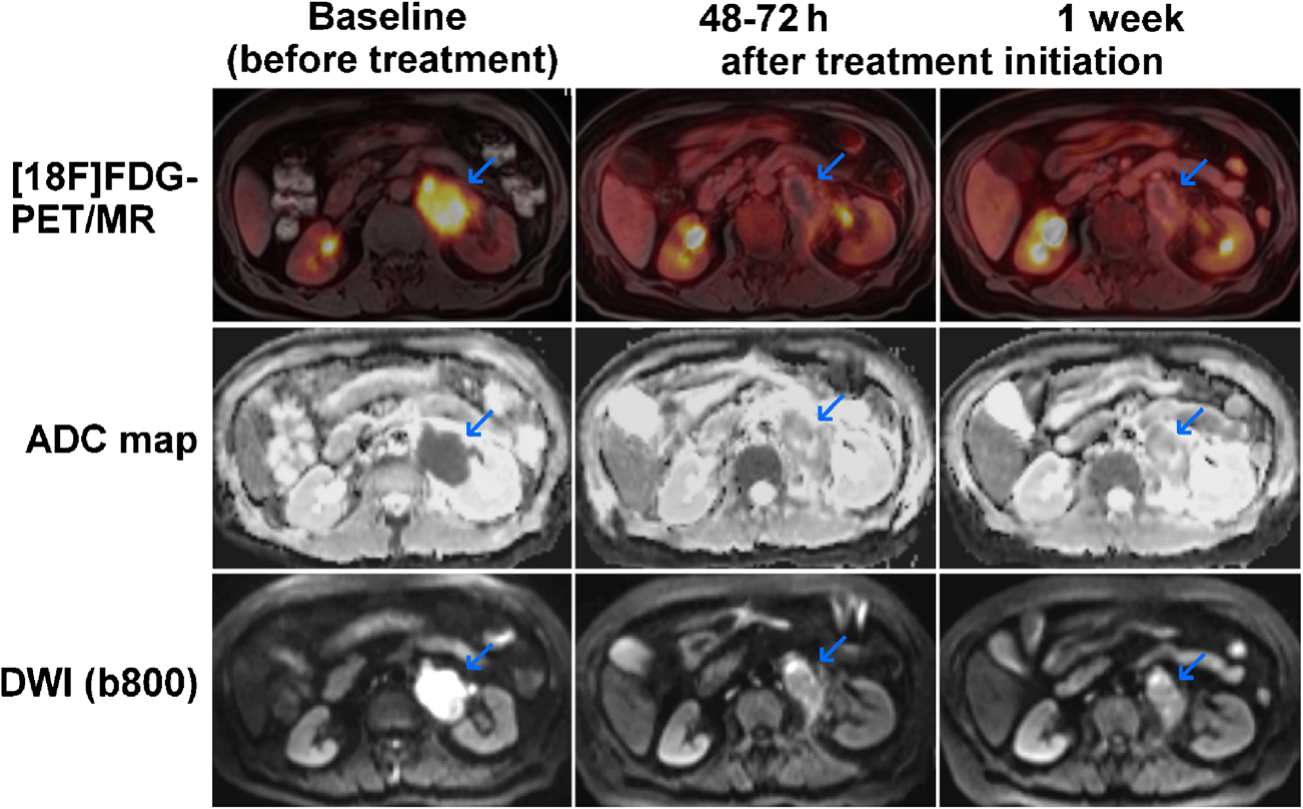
A 68-year-old woman with DLBCL of the left adrenal gland (*blue arrows*). The lymphoma shows intense glucose metabolism (high [^18^F]FDG uptake; high SUVs) at baseline on the axial fused [^18^F]FDG PET/MR image, and a clear diffusion restriction on the DWI image (high signal) and the ADC map (low signal). The PET/MR image at 48–72 h after treatment initiation (R-CHOP) shows an almost complete reduction in [^18^F]FDG uptake (reduced glucose metabolism) and a clear increase in tissue diffusivity (increased ADC, indicating reduced cell density) at this time-point. The PET/MR image at 1 week after treatment initiation shows an additional moderate decrease in lesion size relative to the baseline image
